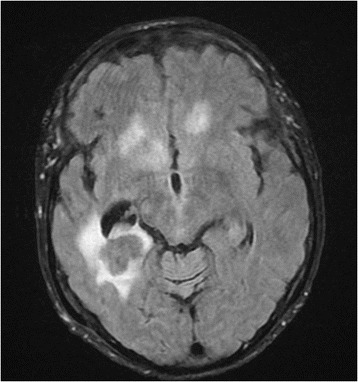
MRI at time of progression 4 weeks later
